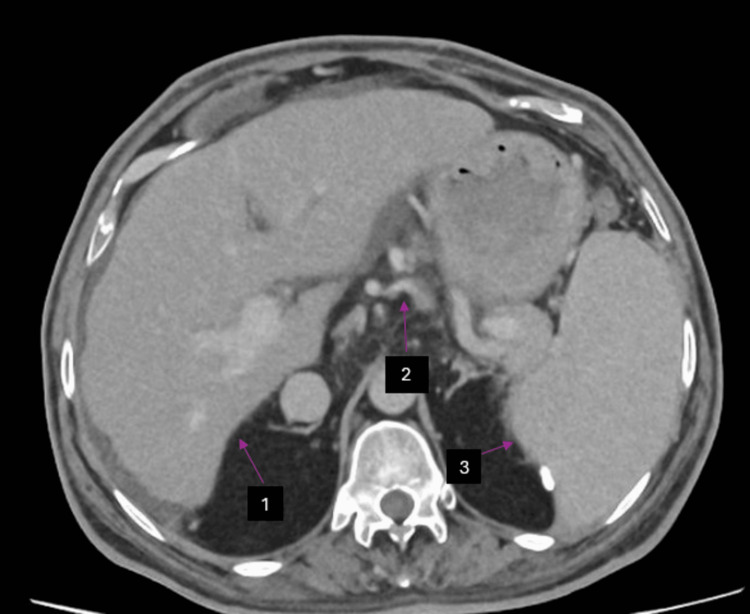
CT abdomen and pelvis Arrows indicating (1) cirrhotic liver, (2) collaterals indicating portal hypertension, and (3) splenomegaly
